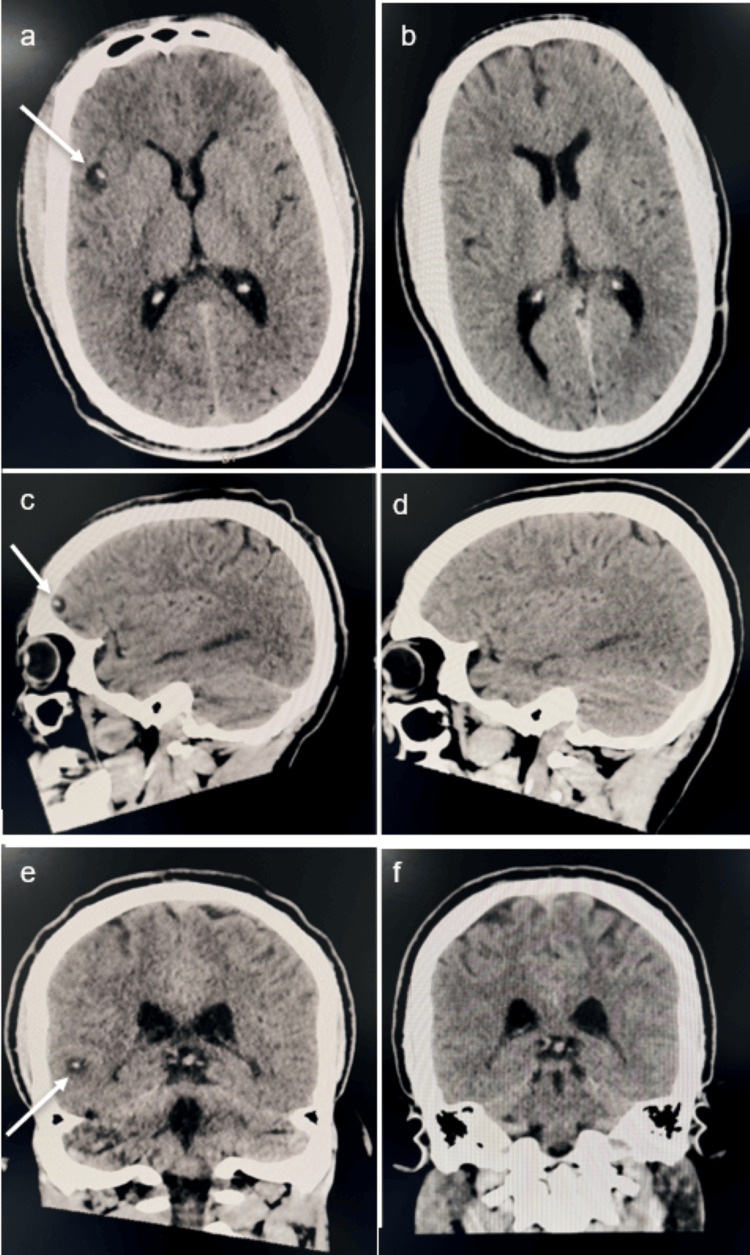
Nonenhanced computed tomography scan of the head showing significant regression of the multiple cystic lesions with a central scolex, as marked by the arrows, where a, c, e represent the lesions before anthelmintic drugs, and b, d, f show the regression of lesions after anthelmintic drugs, in the a-b) Axial, c-d) Sagittal, through left hemisphere, and e-f) Coronal views.
